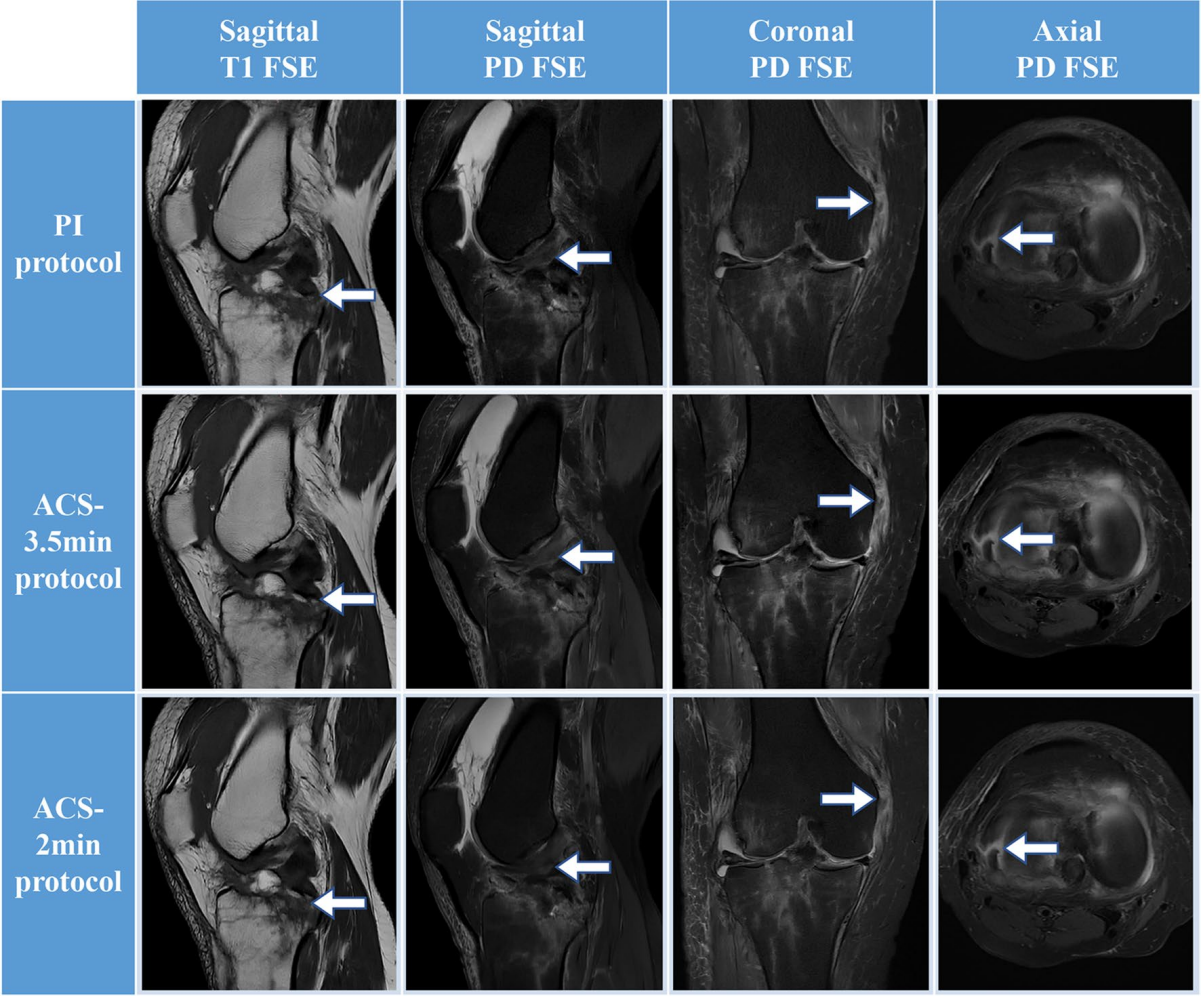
MRI images of a 50-year-old woman with acute trauma. From left to right, sagittal T1-weighted, proton density (PD)–weighted fat-suppressed sagittal, coronal, and axial images of fast spin-echo (FSE) imaging, respectively. Sagittal T1 image shows tibial plateau fracture (arrows); sagittal PD image shows anterior cruciate ligament injury (arrows) and bone marrow edema, joint cavity effusion, and synovial hyperplasia; coronal PD image shows medial collateral ligament tear (arrows); transverse axial view shows medial meniscus tear (arrows)
